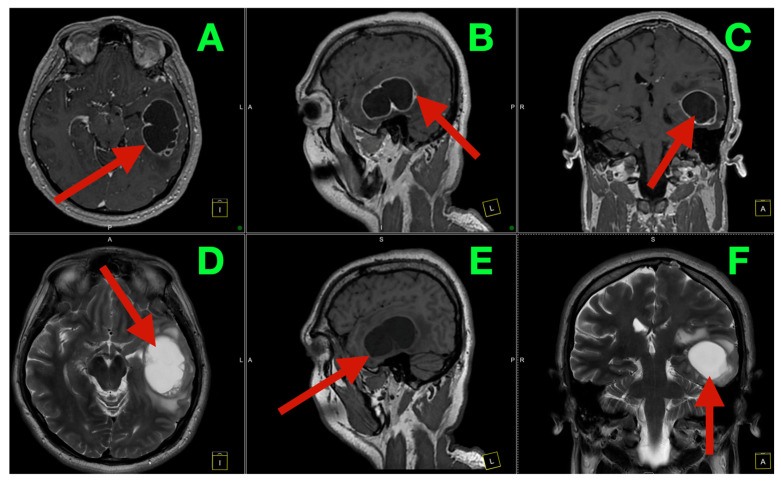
Preoperative MRI of the dominant left temporo-basal cystic tumor. (A) Axial T1 post-contrast: large left temporo-basal multiloculated cystic mass (arrow) with thick, irregular rim enhancement, enhancing septations, and focal mural nodularity, deforming the basal temporal cortical ribbon (inferior temporal gyrus/temporal pole region), concordant with progressive expressive aphasia and cue-resistant anomia. (B) Sagittal T1 post-contrast: skull base–adjacent footprint (arrow) along the middle cranial fossa floor with inferior temporal mass effect. (C) Coronal T1 post-contrast: enhancing mural interfaces (arrow) near the perisylvian corridor/Sylvian fissure, topographically aligned with dominant temporo-perisylvian language output circuitry. (D) Axial T2: hyperintense cystic compartments (arrow) with perifocal T2 hyperintensity tracking into temporo-subcortical associative white matter. (E) Sagittal T1 non-contrast: volumetric cystic component (arrow) defining the inferior temporal surgical corridor toward the skull base. (F) Coronal T2: surrounding parenchymal signal abnormality extending toward the insular–Sylvian region along the expected course of MCA M2–M3 opercular branches, supporting network-level disruption beyond the cyst wall.
